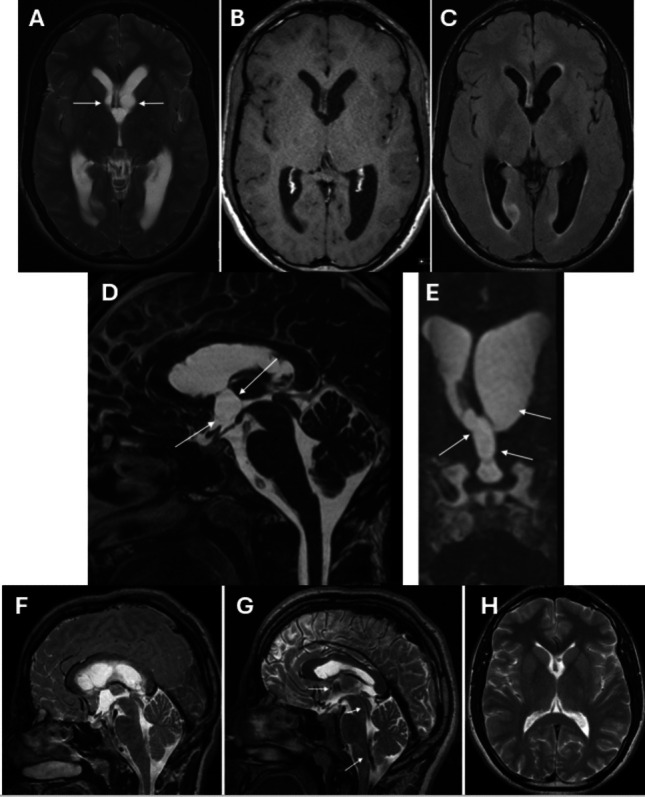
A, B and C—Pre-operative axial T2 (1A), T1 (1B) and FLAIR images (1C) demonstrate the cystic lesion involving both Foramina of Monro (arrows) and projecting into the third ventricle with ensuing hydrocephalus. Note that the lesion is isointense to CSF on all sequences. D and E—Mid-sagittal FIESTA image (1D) demonstrates the 3rd ventricular component of the cyst (arrows). Coronal reformat (1E) shows the cyst involving both foramina of Monro (larger on the left) and third ventricle (arrows). F and G—Mid-sagittal T2 SPACE image (1G) following cyst resection shows normalisation of CSF flow across the ventricular system with flow voids present across the foramina of Monro, aqueduct and fourth ventricular outlet foramina (arrows) that were absent or reduced on the corresponding pre-operative 2D multi-slice T2w FSE image (1F). H—Axial T2 image from 3.5 years follow-up MRI shows normal ventricular size with no recurrence of the cyst. (The tip of a ventricular catheter is visible in the left lateral ventricle)
